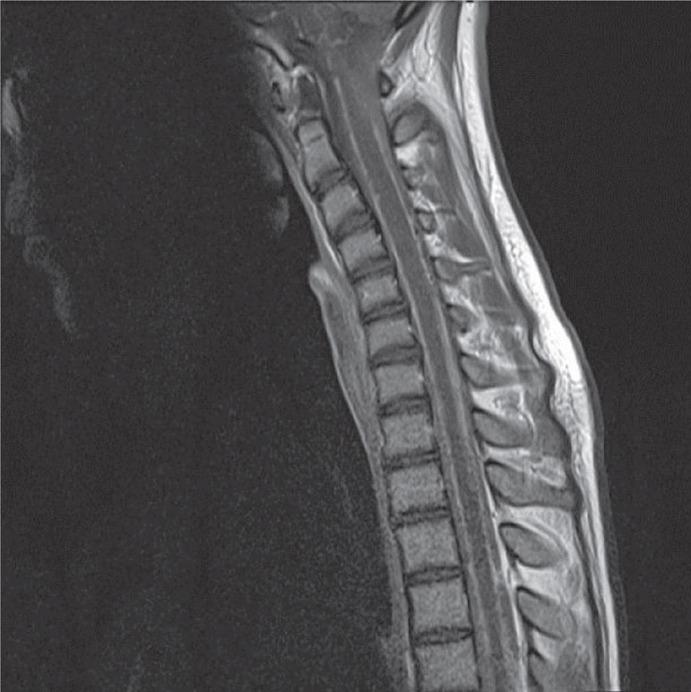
Spinal magnetic resonance imaging (MRI) on T1-weihgted demonstrated diffuse enhancement after intravenous gadolinium injection in cervical
